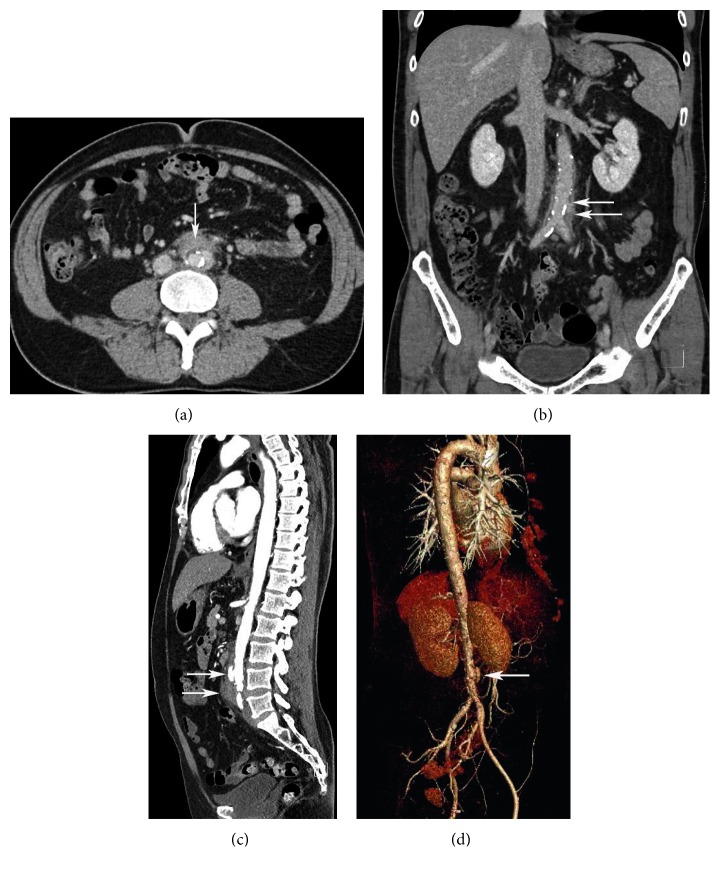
(a) Transverse view of abdominal CT shows periaortic soft tissue thickening adjacent to aorta (arrow). (b) Coronal view of abdominal CT without IV contrast shows disruption of calcification in aortic wall (upper arrow) and periaortic soft tissue thickening around the infrarenal aorta and around bifurcation of aorta into common iliac arteries (lower arrow). (c) Sagittal view of abdominal CT, with IV contrast, shows pseudoaneurysm formation (upper arrow) and periaortic soft tissue thickening around the infrarenal aorta (lower arrow). (d) Reconstructive CT angiogram shows pseudoaneurysm formation (arrow).
